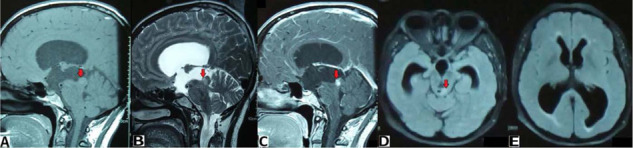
Preoperative brain MRI. (A) Sagittal tbl1 WI, (B) sagittal tbl2 WI, (C) sagittal tbl1 WI injected, (D) axial FLAIR passing through the Sylvius aqueduct, (E) axial FLAIR passing through the lateral ventricles’ frontal horns. The lesion in the tectum appears isointense on tbl1 WI and hyperintense on tbl2 WI and FLAIR images, with remarkable enhancement after gadolinium injection (arrow). The lesion caused an obstructive hydrocephalus (E).
